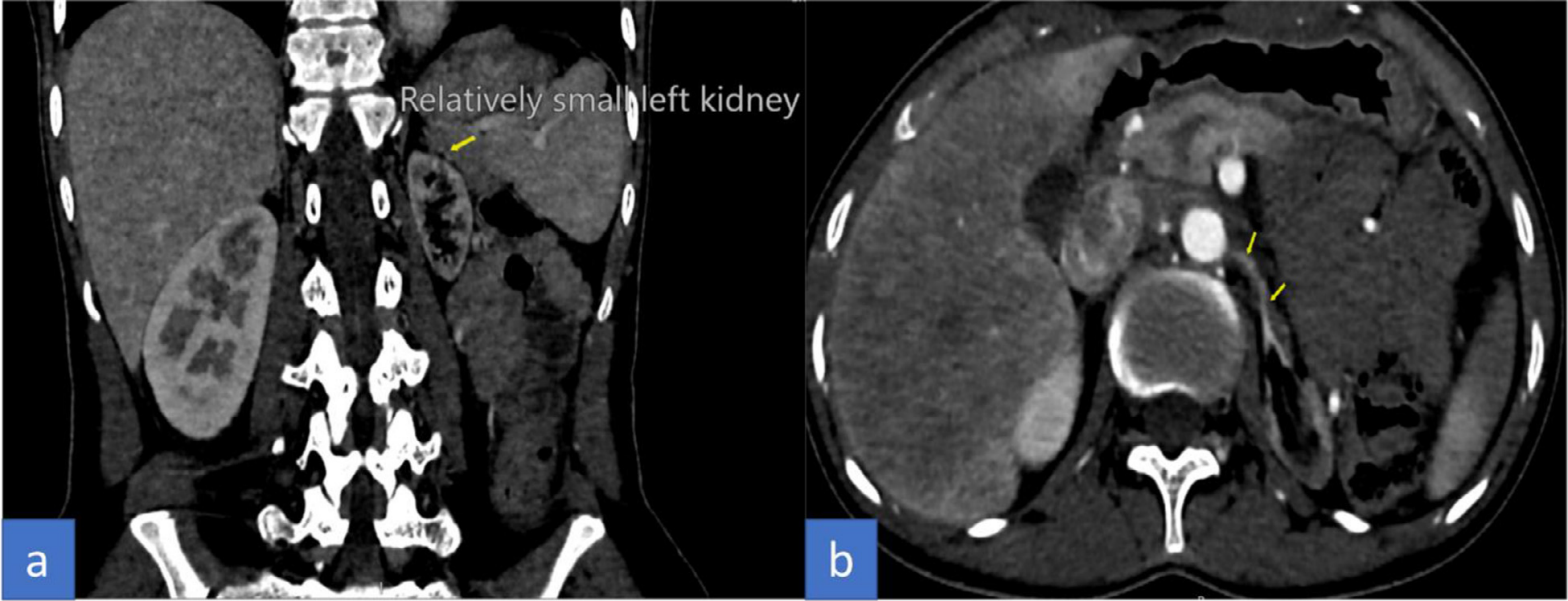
CTA coronal view (A), axial view (B) showing small left kidney with marked thickening of the left renal artery, narrowing of its lumen, and poor opacification of the contrast (arrow).
